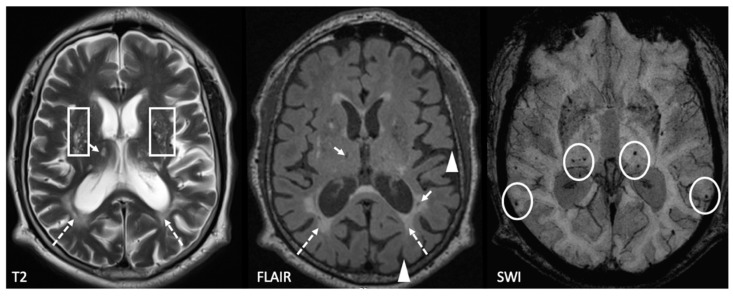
Example of characteristic SVD MRI features: dotted arrows—white matter hyperintensities (WMH), solid arrows—lacunes, circles—microbleeds, triangles—sulcus enlargement as a marker of brain atrophy, and rectangles—multiple enlarged perivascular spaces.
